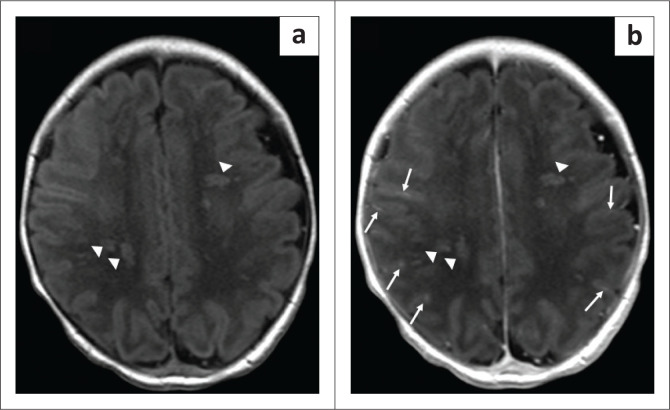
Axial T1-weighted MRI of the brain at the level of centrum semiovale (repetition time msec/echo time msec, 300/2.52, 3-mm section thickness), unenhanced (a) and contrast-enhanced (b) after intravenous injection of 1 mL of gadoteridol (ProHance; Bracco). There were areas of non-enhancing signal abnormality in the bilateral supratentorial frontal white matter (solid white arrow heads on images [a] and [b]), corresponding to the restricted diffusion. Subtle leptomeningeal contrast enhancement seen (solid white arrows on image b).
